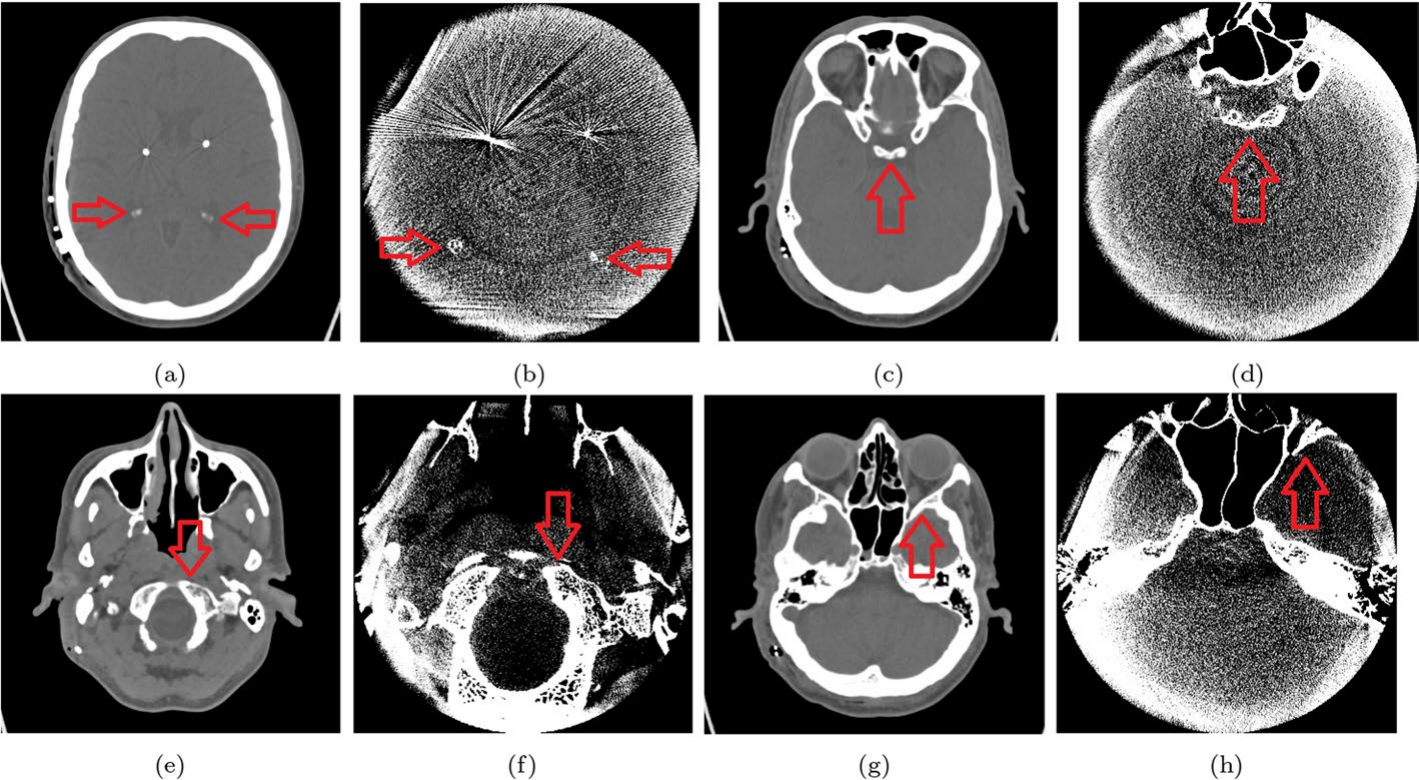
Overview of the crucial anatomical structures corresponding in both modalities: CT and DCTm images. These points are used for initiating and evaluating registration with input rater, but not the same points that were used for initialization are used for computing the target registration error (TRE). ((1) and (2)): bilateral calicifications in choroid plexus (not apparent in all patients), ((3) and (4)): clivus/tuberculum sellae, ((5) and (6)): atlantoaccipital joint, ((7) and (8)): superior orbital fissure
